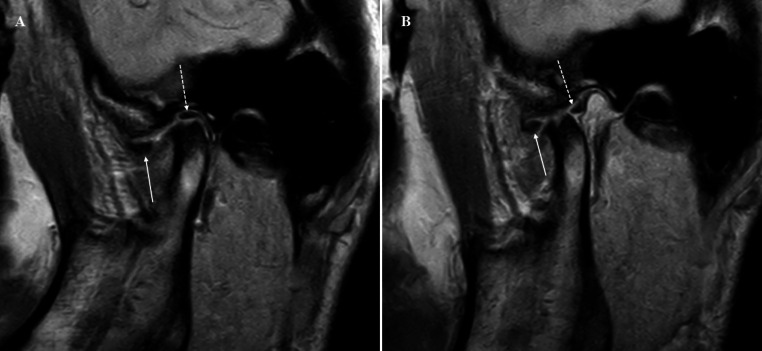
Modified-sagittal proton density-weighted MR images with closed mouth (A) and open mouth positions (B), representing disc perforation of the left temporomandibular joint (patient #15). Interruption in the intermediate zone of the disc with separation of the anterior (arrow) and posterior (dashed arrow) bands is noted
